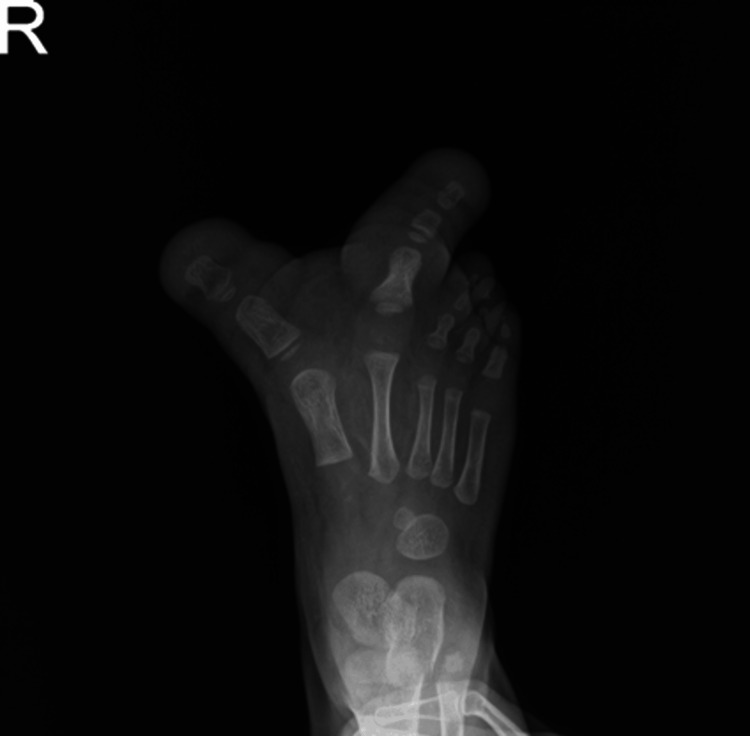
X-ray of the right foot before the operation
